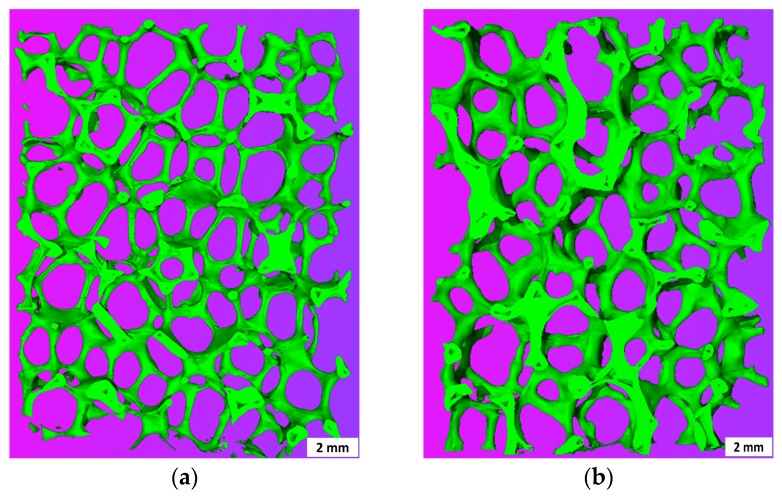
µ-CT 3D reconstruction of 3 mm thick slices of (a) ZTA16-70 and (b) ZTA20-80.
